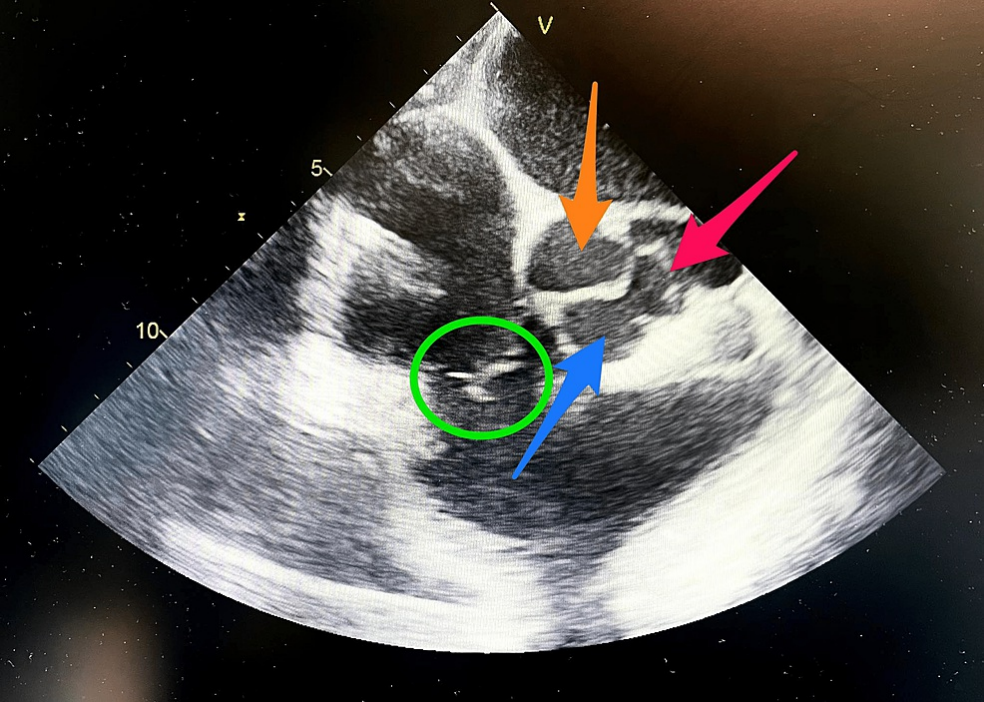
Transesophageal echocardiogram at 60 degrees. Short axis view of aortic valve shows perforation and rupture of left coronary leaflet. Green circle shows the tricuspid valve. Blue arrow shows the right coronary cusp of aortic valve. Pink arrow shows left coronary cusp of aortic valve with teared left coronary leaflet. Orange arrow shows non-coronary cusp of aortic valve.
